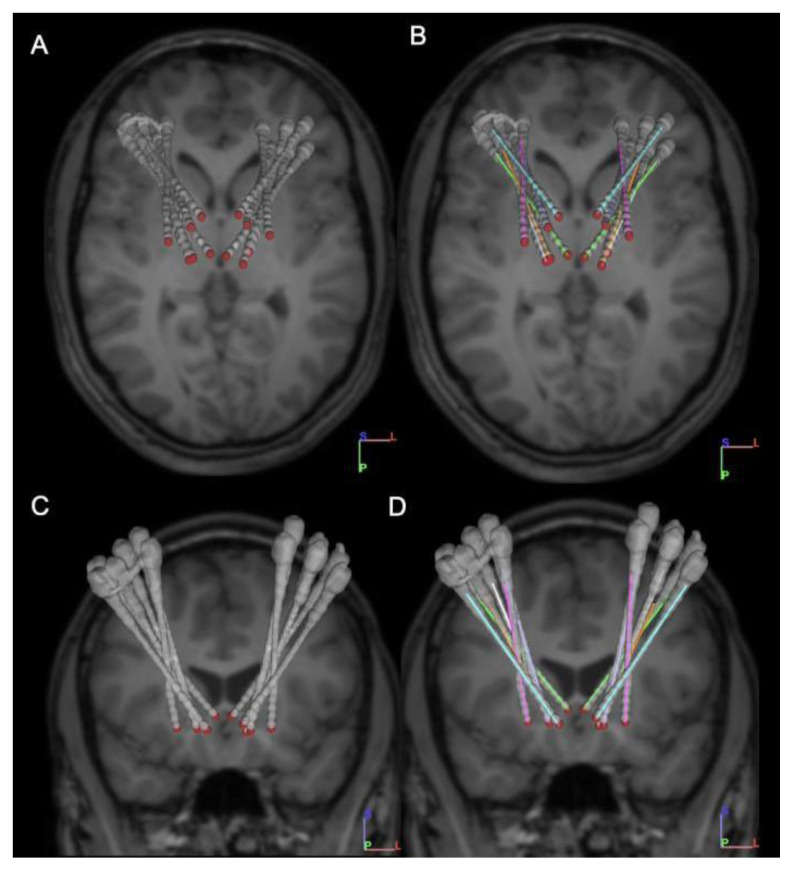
Axial (A,B) and coronal (C,D) views of the postoperative CT overlaid on the preoperative MRI, showing the lead locations for the Adtech stereo EEG leads.
